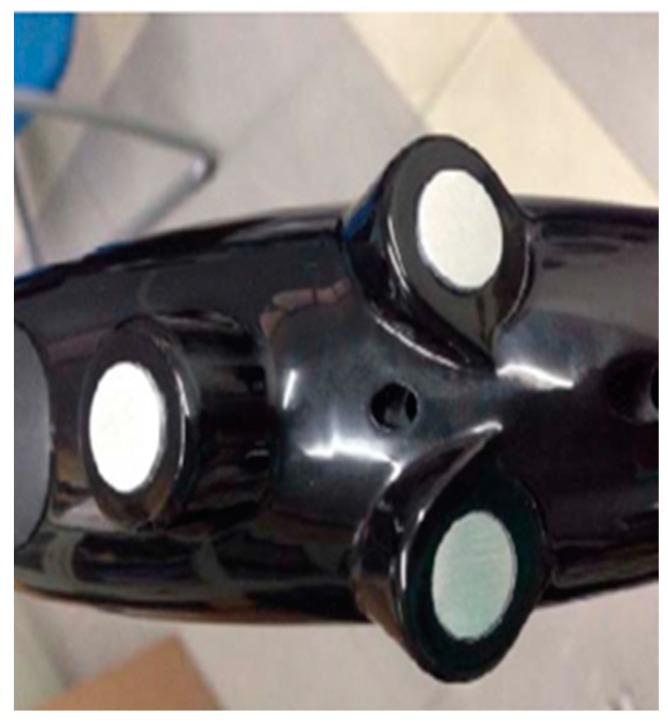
Replacement of three ultrasonic sensors on the cane [54].
